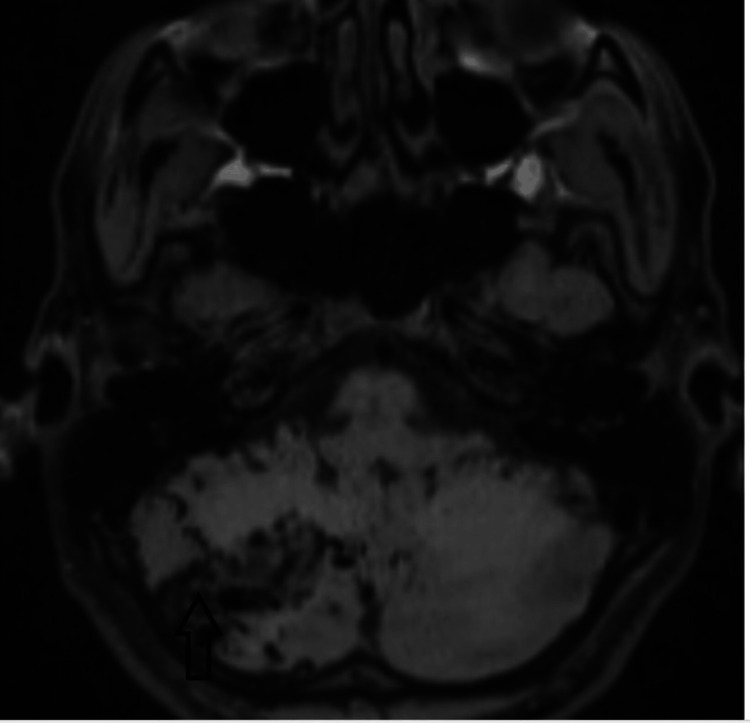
Incidental right cerebellar hemispheric hemangioma found on an MRI head scan study.
